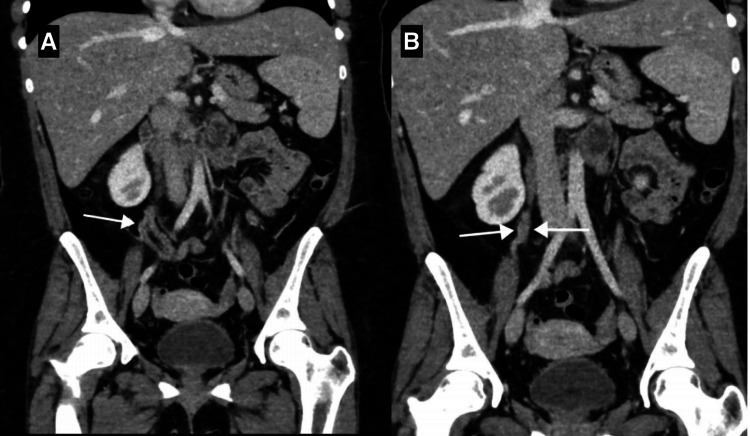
(A) CT scan (coronal view, porto-venous phase) showing dilated tortuous right ovarian vein with subtle stranding, suggesting thrombosis (white arrow). (B) CT scan (coronal view, venous phase) showing dilated right ovarian vein (white arrows). CT: computed tomography
